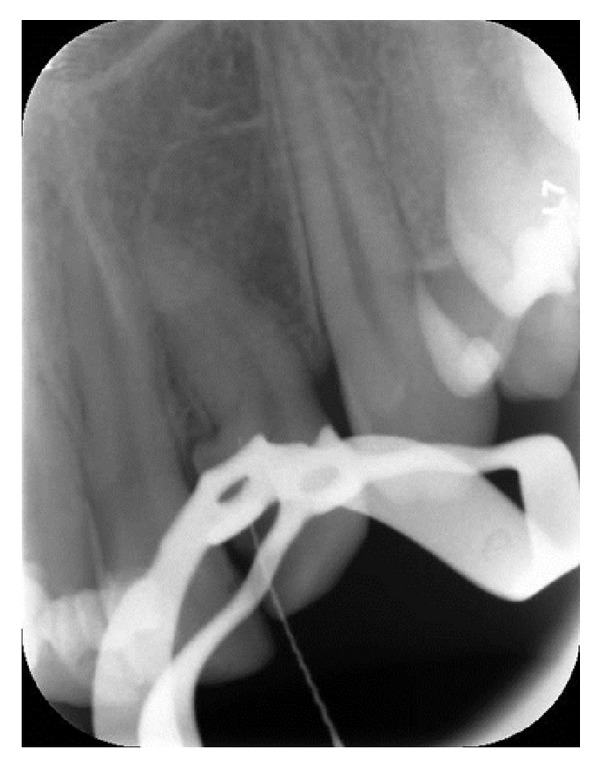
Radiograph showing the fusion of the main canal and invagination.
